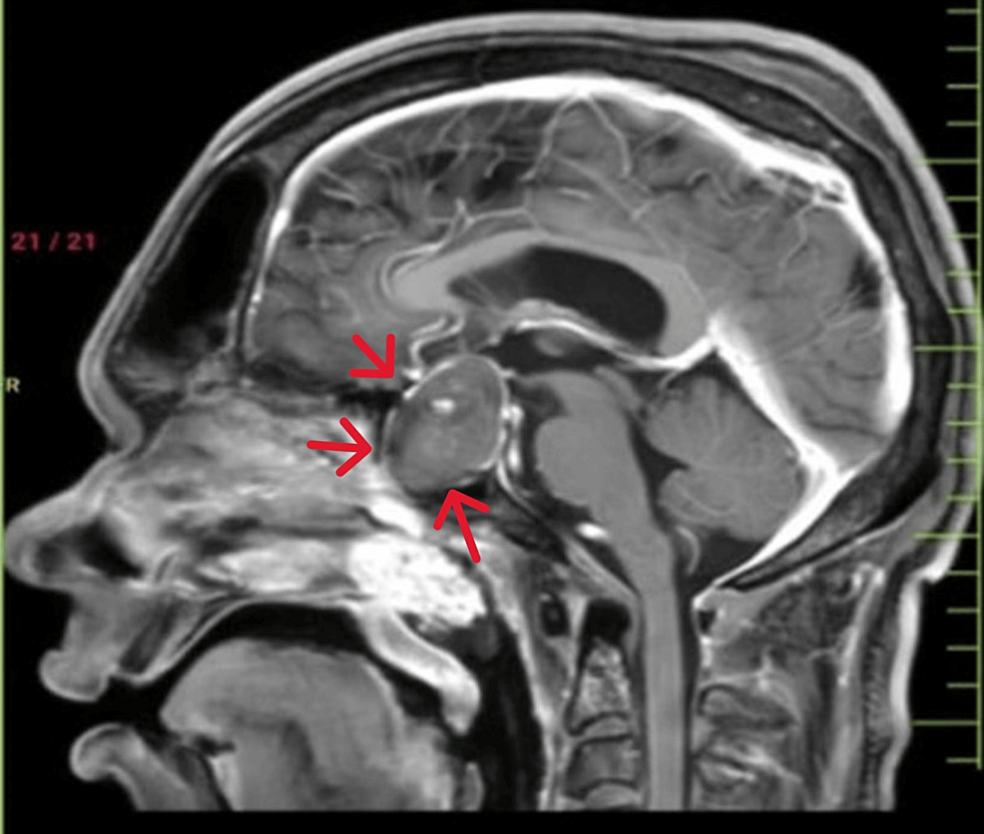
Contrast-enhanced MRI (CE-MRI) Brain showing heterogenous enhancement with altered signal intensity was observed in the sellar and suprasellar region, approximately measuring 4x3.7×3cm pituitary macroadenoma (red arrow)
